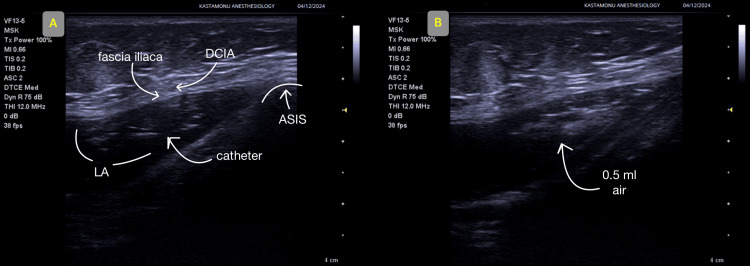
Ultrasound images. (A) continuous suprainguinal fascia iliaca block placement; (B) confirmation of catheter position. The same method was applied in both cases. DCIA: deep circumflex iliac artery, LA: local anesthetic solution, ASIS: anterior superior iliac spine.
